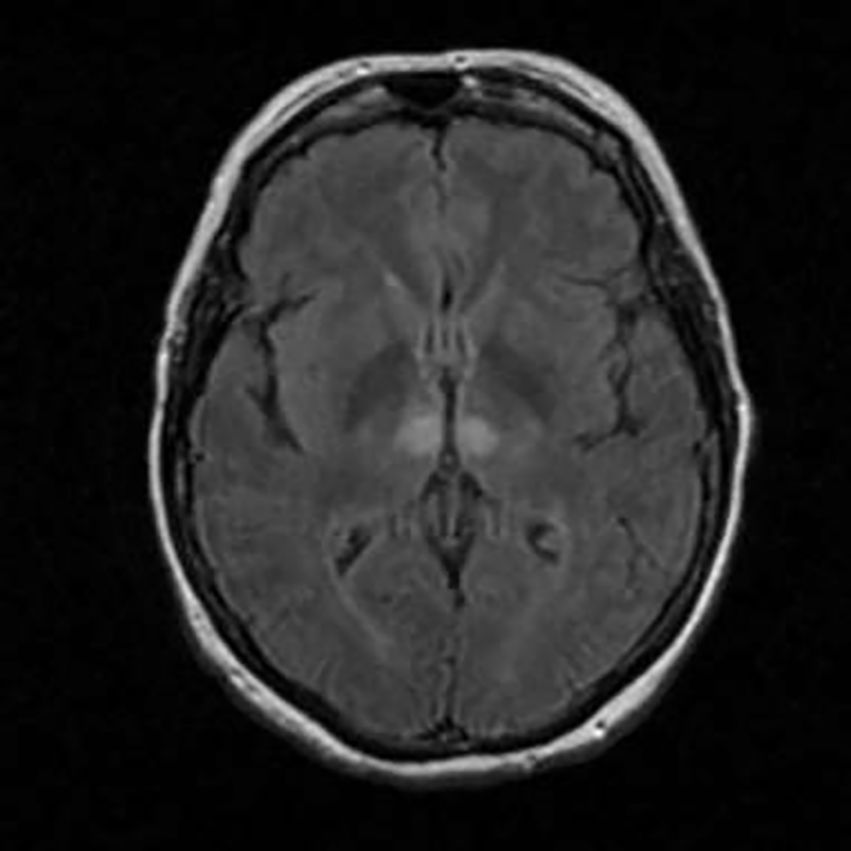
T1/FLAIR signal weighed axial MR demonstrating hyperdensities of bilateral paramedian thalami.
